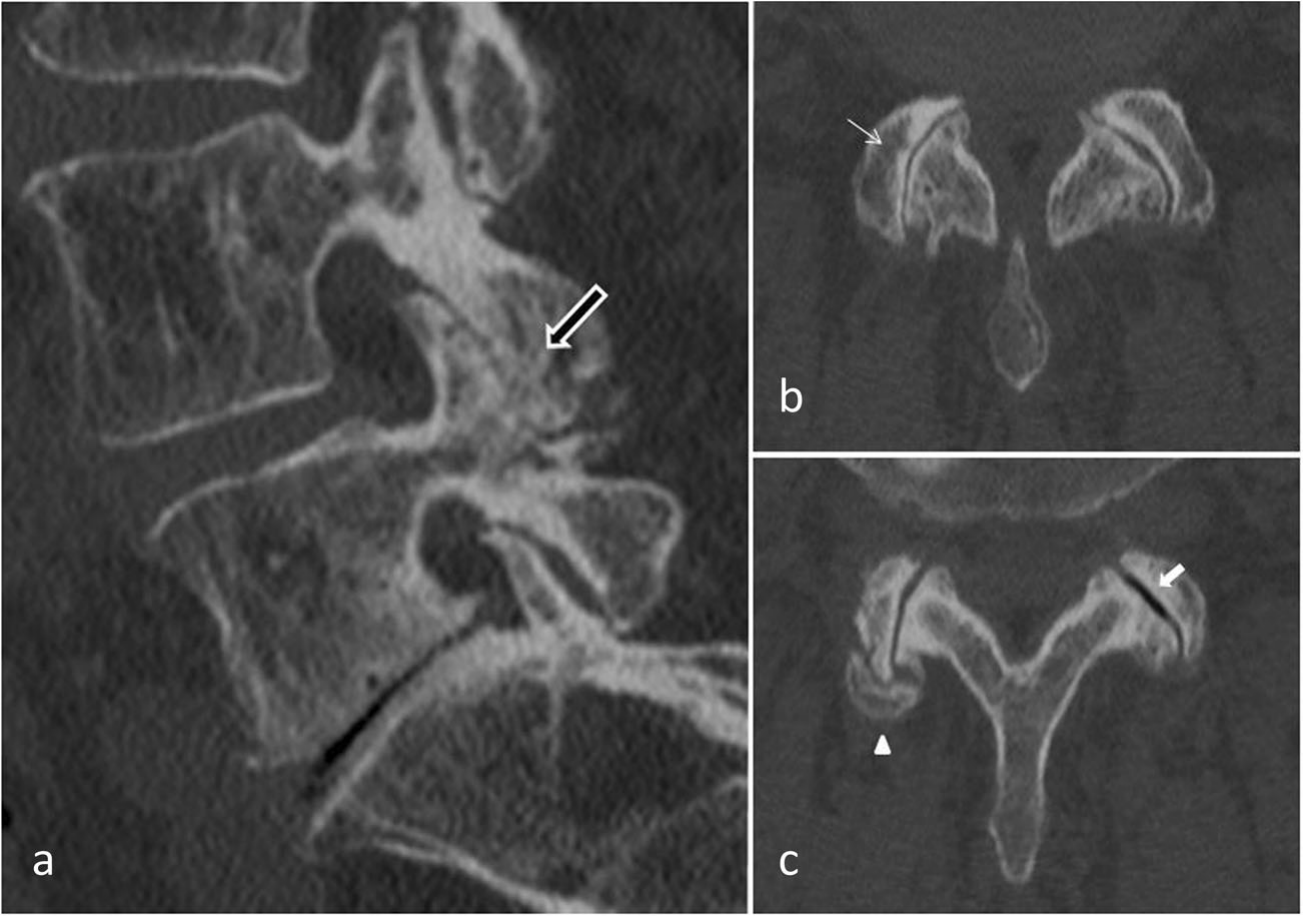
Degenerative facet joint osteoarthritis (FJOA): Sagittal (a) and axial (b, c) CT views. Hypertrophy of the posterior articular process (black arrow). Joint space narrowing (thin white arrow). Joint capsule calcification (arrow head) and vacuum phenomenon (white arrow)
